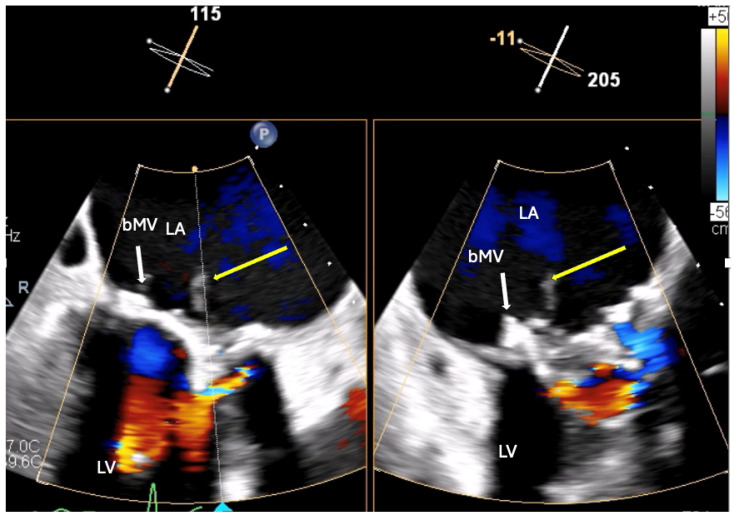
Transoesophageal echocardiography image—cross-sectional image of degenerated immobile mitral valve bioprosthesis (bMV) (yellow arrows indicate vegetation attached to mitral valve). LV: left ventricle; LA: left atrium.
